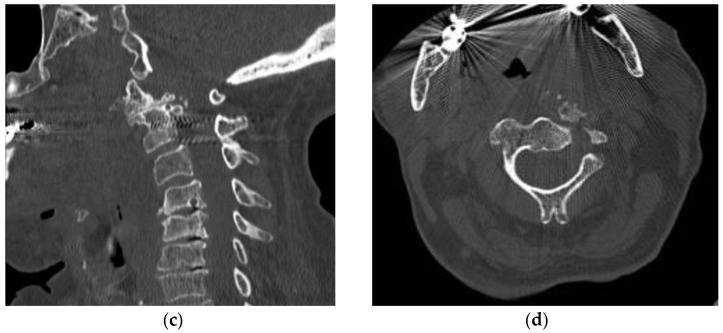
Preoperative (a,b) and postoperative (c,d) CT scans of patient 2, documenting a hyperostotic bone mass with three components involving C1 and the left C2 lateral mass. The component along the midline was resected obtaining a satisfactory reduction in the compression on the posterior pharyngeal wall.
